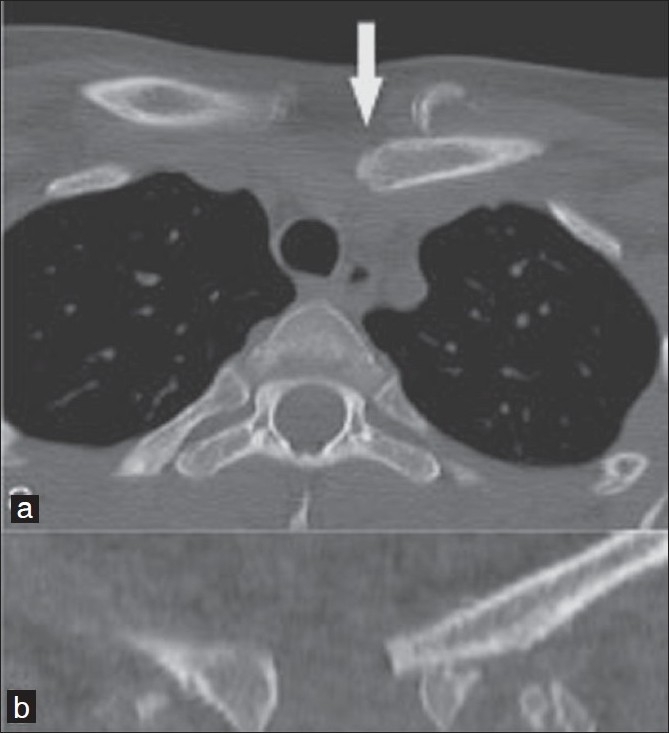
(a,b) Axial CT at the clavicular level showing a medial and posterior displacement of the medial end of the left clavicle (solid arrow) and revealing a medial fracture of the clavicle
